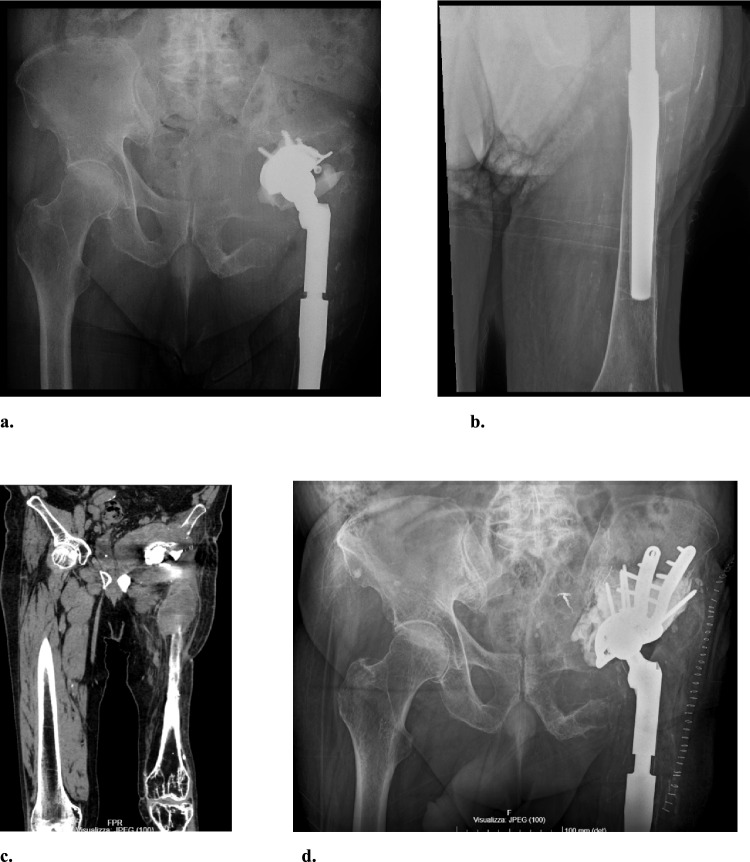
a–d A 76-year-old male presented with a painful left limb mass. Coronal CT showed a large pseudotumor in intra- and extrapelvic regions with extensive osteolysis. The cup was loose (Paprosky 3B), but the femoral stem remained stable. Metal-on-Polyethylene bearings were used, with negative metal ion levels. The PCS classification categorized the case as Psi C3 S1. Treatment included preoperative embolization, mass debulking, cup revision with a cage and cemented dual mobility cup, and head exchange. a Preoperative anteroposterior pelvic x-ray; b Preoperative anteroposterior x-ray of the left thigh, focusing on the distal stem tip; c CT scan OMAR coronal view showing a large pseudotumor with severe osteolysis; d Postoperative anteroposterior pelvic x-ray
